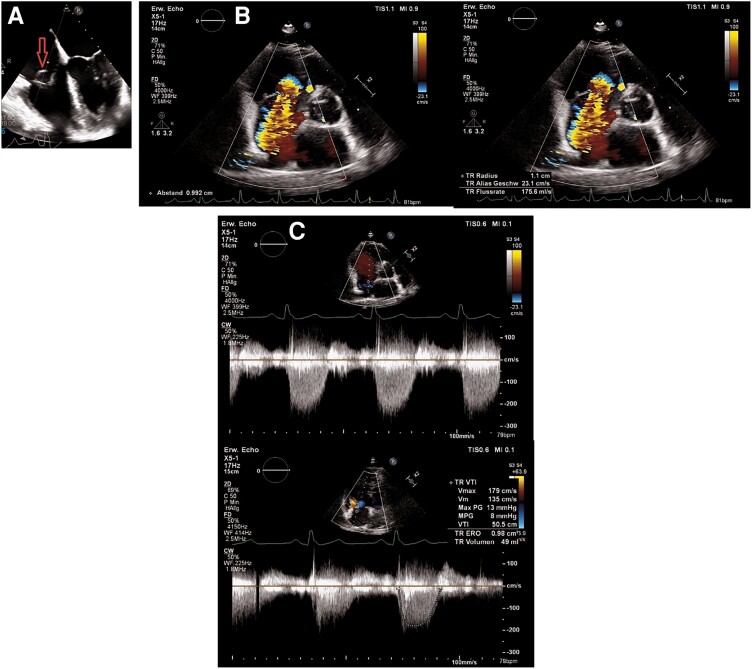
Preoperative transthoracic echocardiography demonstrating torrential tricuspid valve regurgitation. (*A*) Prolapse of the anterior tricuspid leaflet in apical four-chamber view (red arrow). (*B*) Quantitative assessment of tricuspid regurgitation using the proximal isovelocity surface area method. (*C*) The continuous wave Doppler signal of the regurgitant jet is truncated and triangular. Peak 1.7 m/s, effective regurgitant orifice area 0.98 cm^2^, and regurgitant volume 49 mL.
